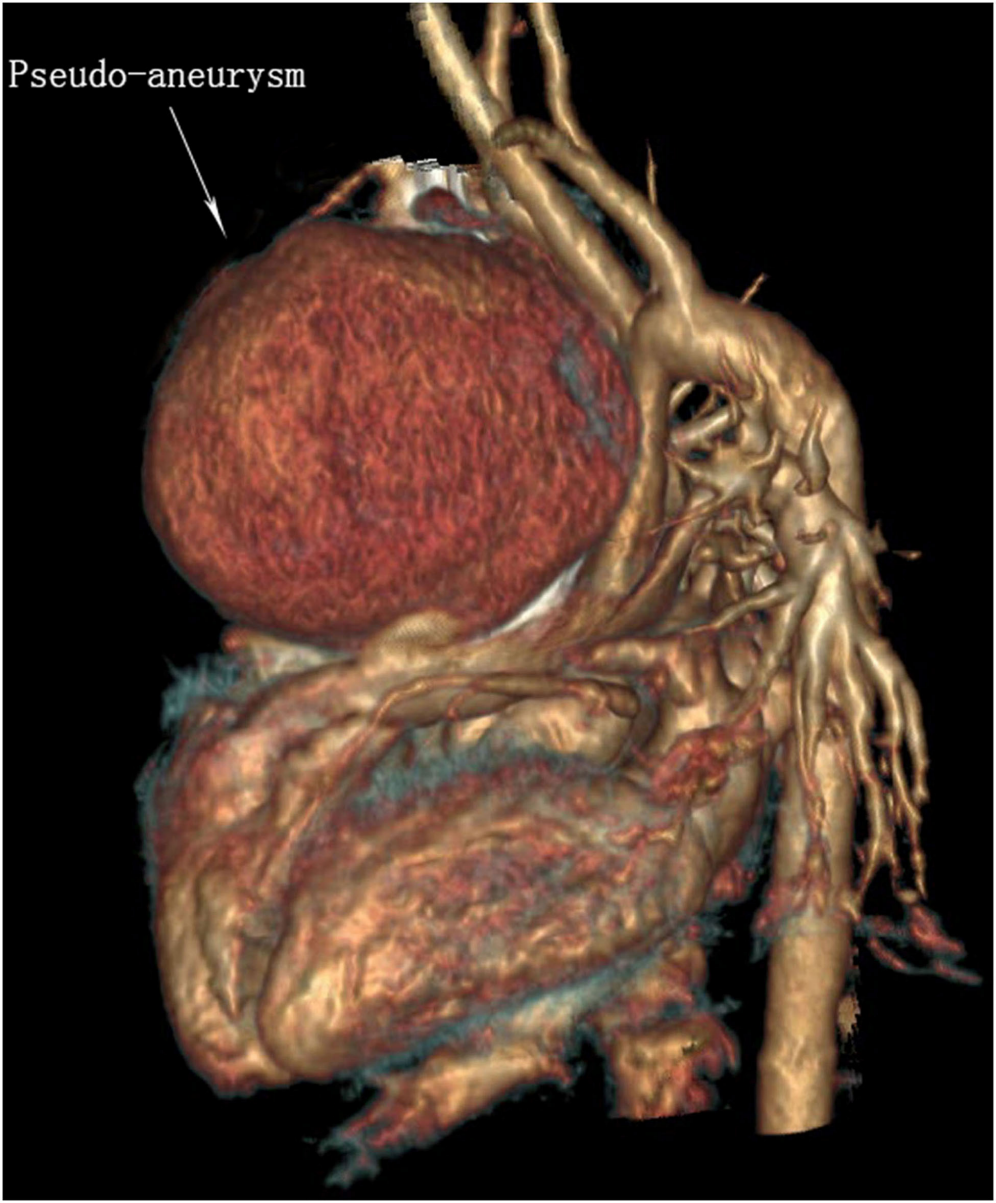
3D reconstruction of sagittal view of contrast enhancement CT image suggests a large pseudo-aneurysm is bound up with the ascending aorta.
